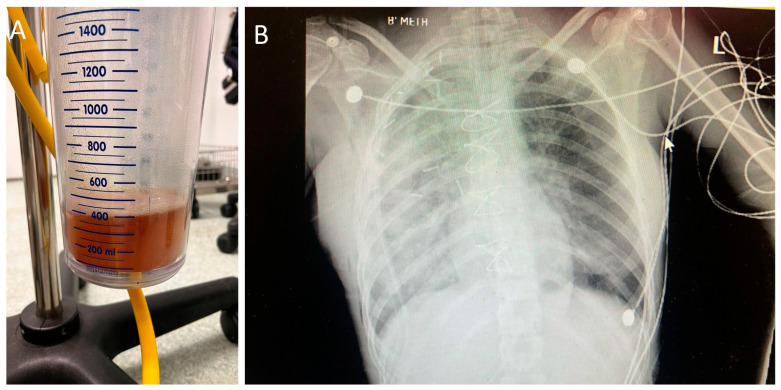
(A) Suction contents; (B) the patient’s postoperative chest X-ray.
